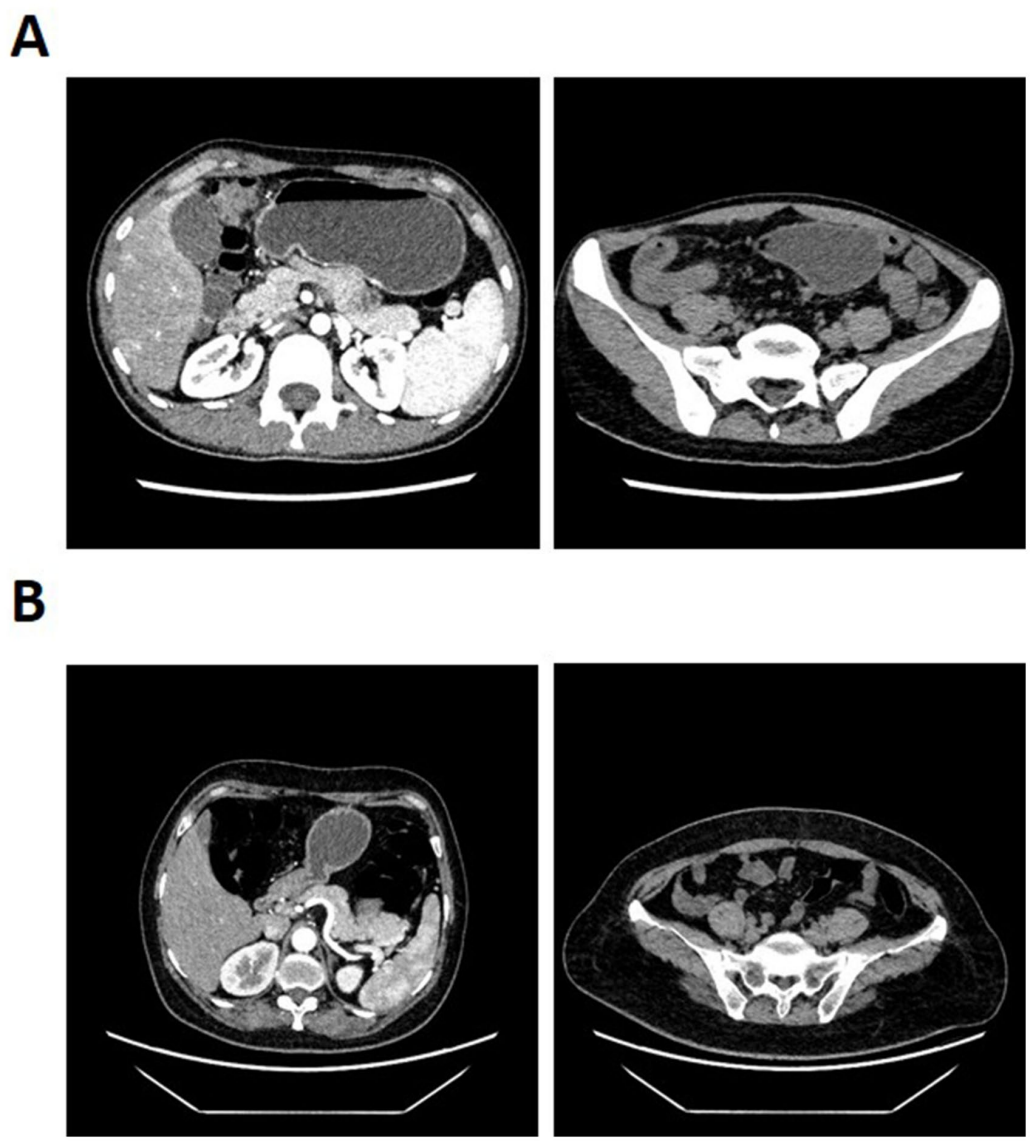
Computed tomography enterography (CTE) images from (A) a CD patient and (B) a healthy control individual.
